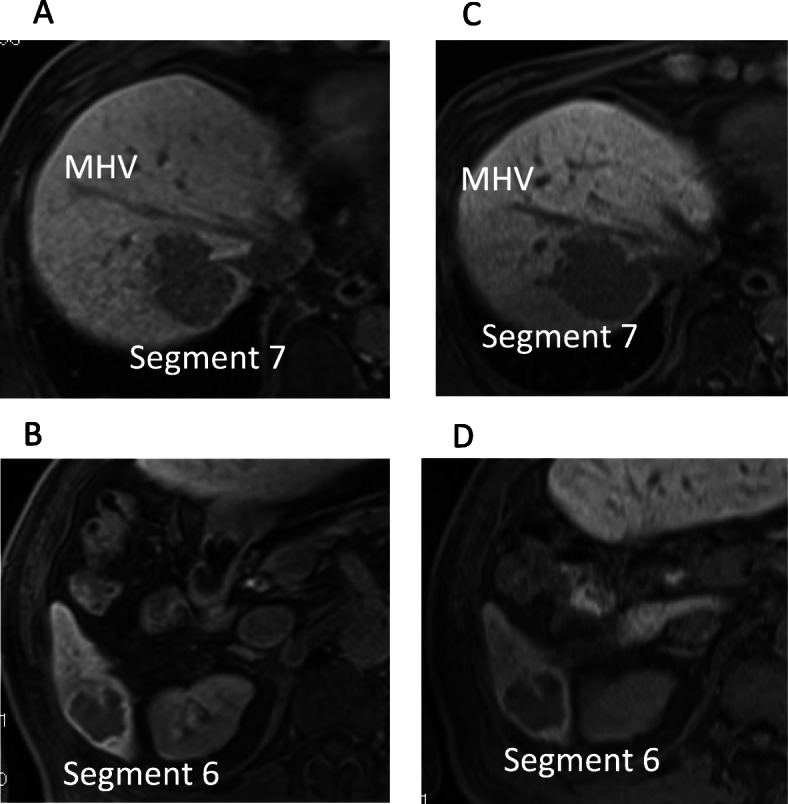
MRI imaging of HCC. a MRI showing the tumor located in segment 7 at the first visit. b MRI shows the tumor located in segment 6 at the first visit. c MRI shows the tumor located in segment 7 before hepatic resection. The tumor grew to 5.1 cm 10 weeks after the first visit. d MRI showing the tumor located in segment 6 before hepatic resection. The tumor grew to 3.1 cm 10 weeks after the first visit. MRI, magnetic resonance image; HCC, hepatocellular carcinoma; CT, computed tomography
